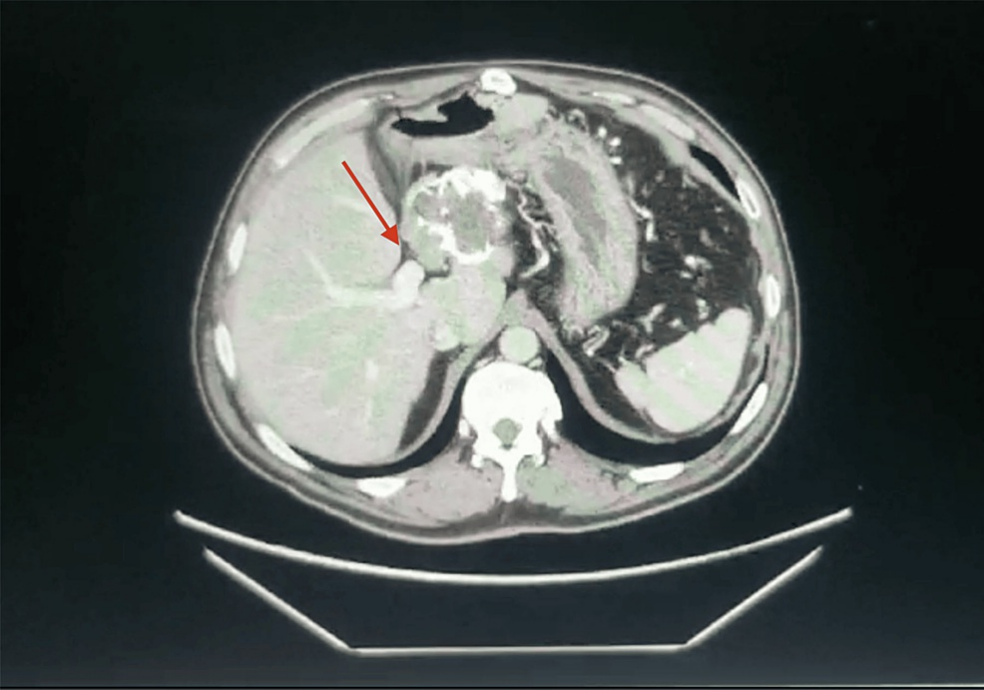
Contrast-enhanced CT scan of the abdomen from 2023. This second view identifies the portal vein in relation to the SPN. SPN, solid pseudopapillary neoplasm
